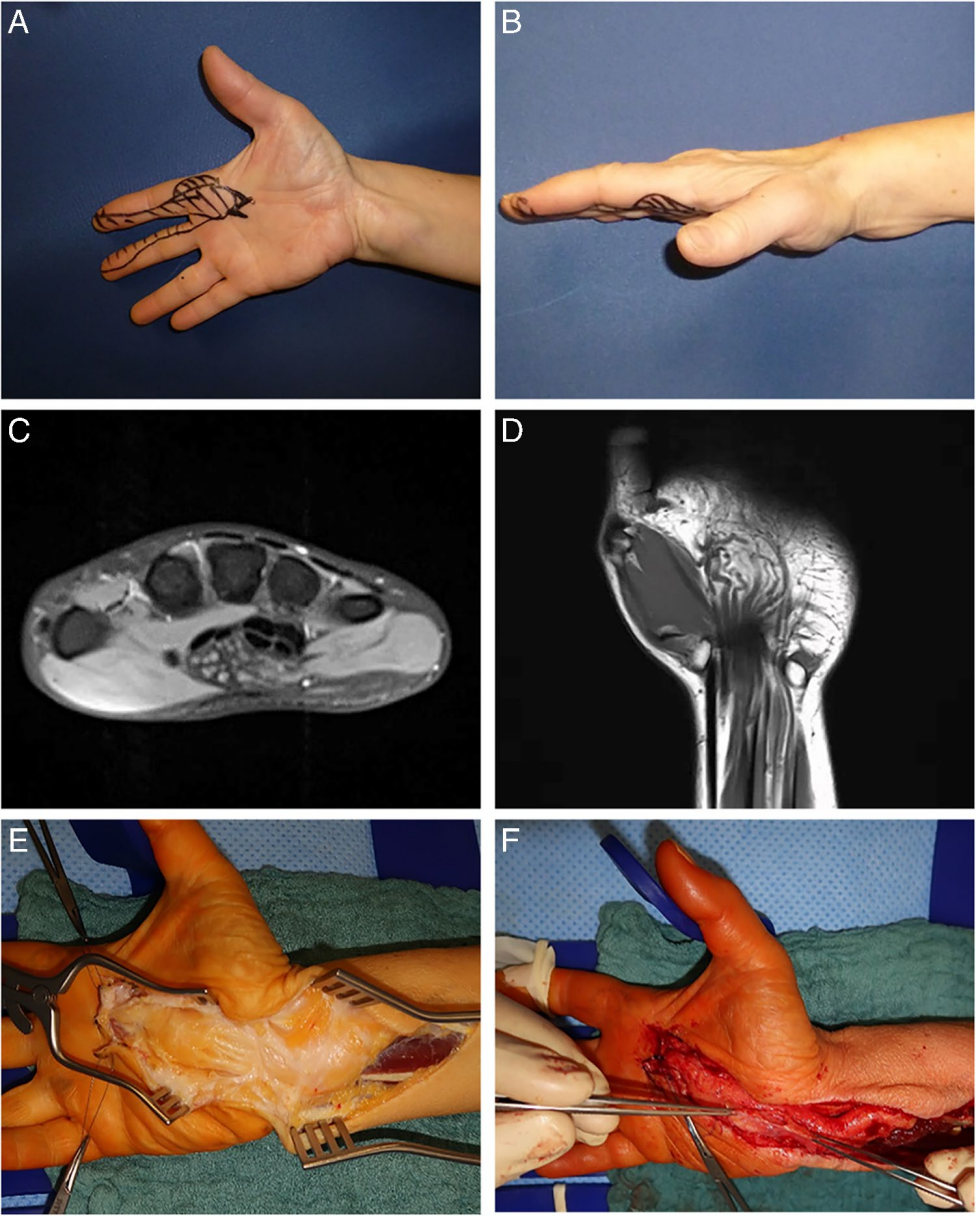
Clinical findings in patients with lipomatosis of the median nerve. A, B Clinical images showing a mass at the wrist. Areas with impairment of sensitivity are marked in black color. C, D MRI images revealing typical features of LPN of the median nerve with spaghetti-like nerve bundles. E, F Intraoperative findings of a giant median nerve at the wrist; the median nerve was covered with a fascial sheet after decompression
